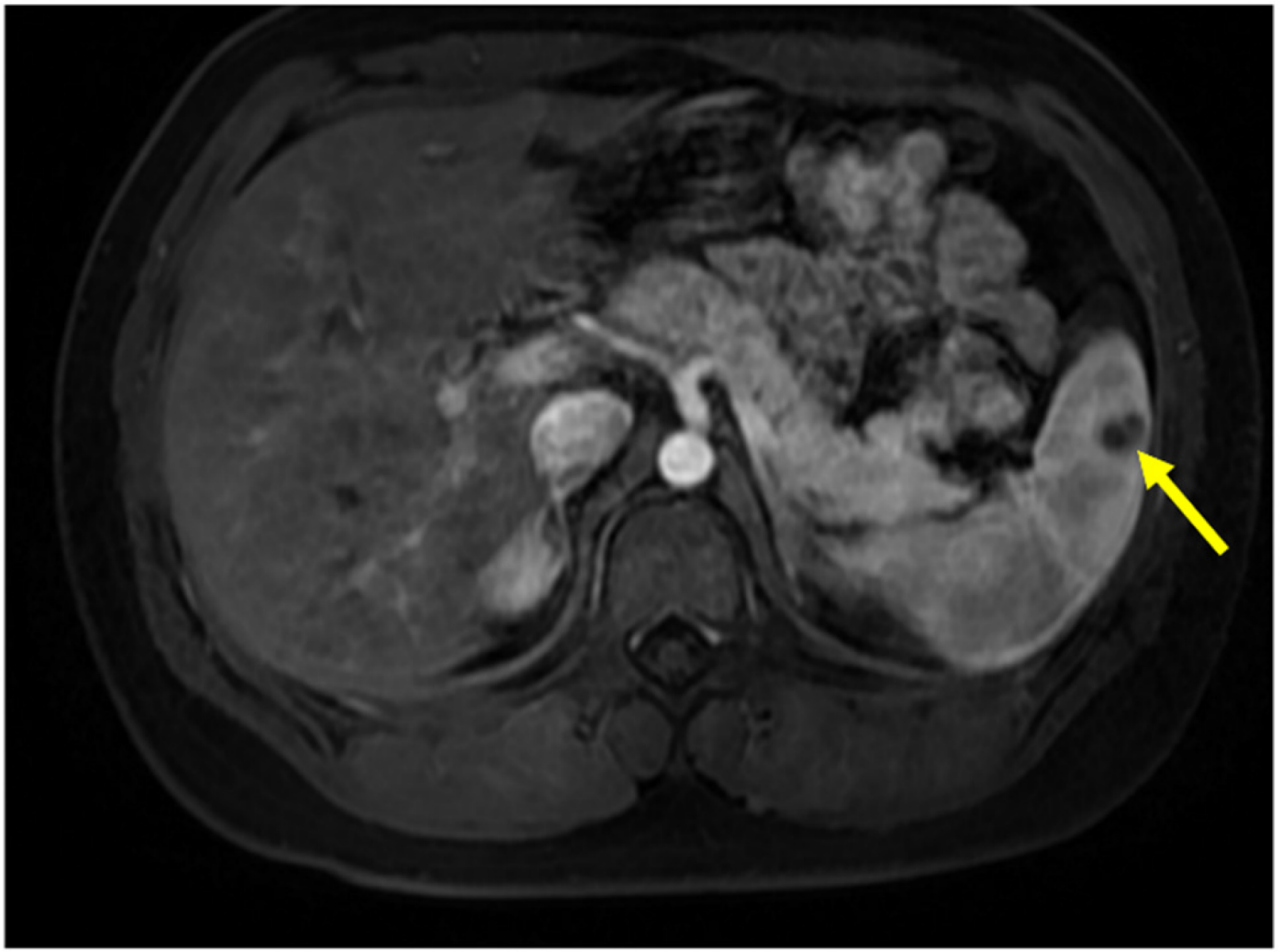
Contrast-enhanced MRI showing a low-density lesion in the spleen (approximately 13 mm).
